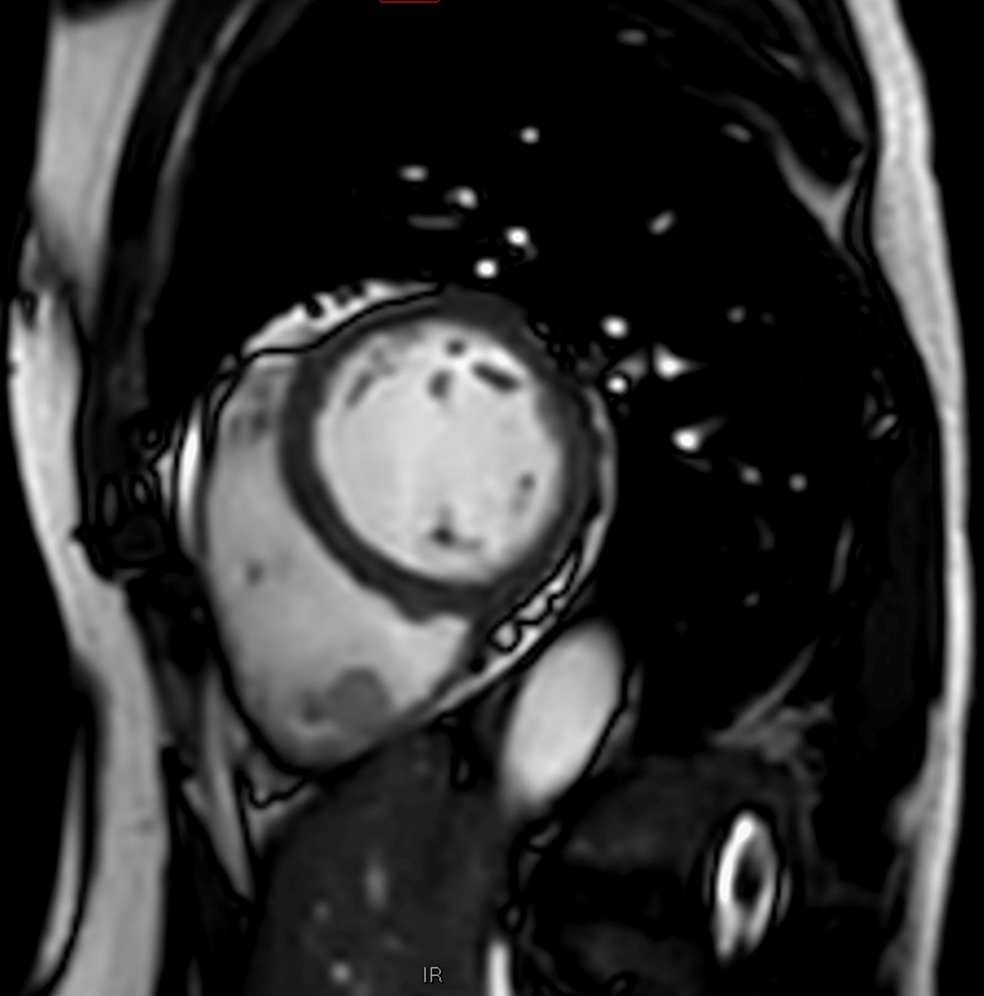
T1-weighted scan of the right ventricular mass
